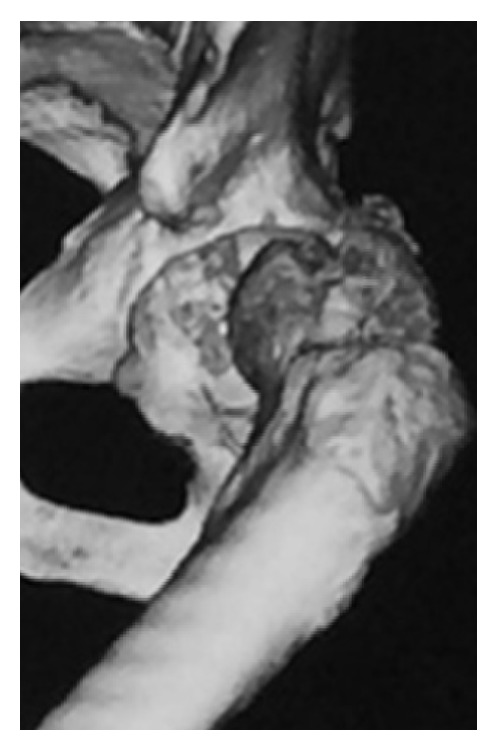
Computed tomography of the left hip joint after redislocation.
